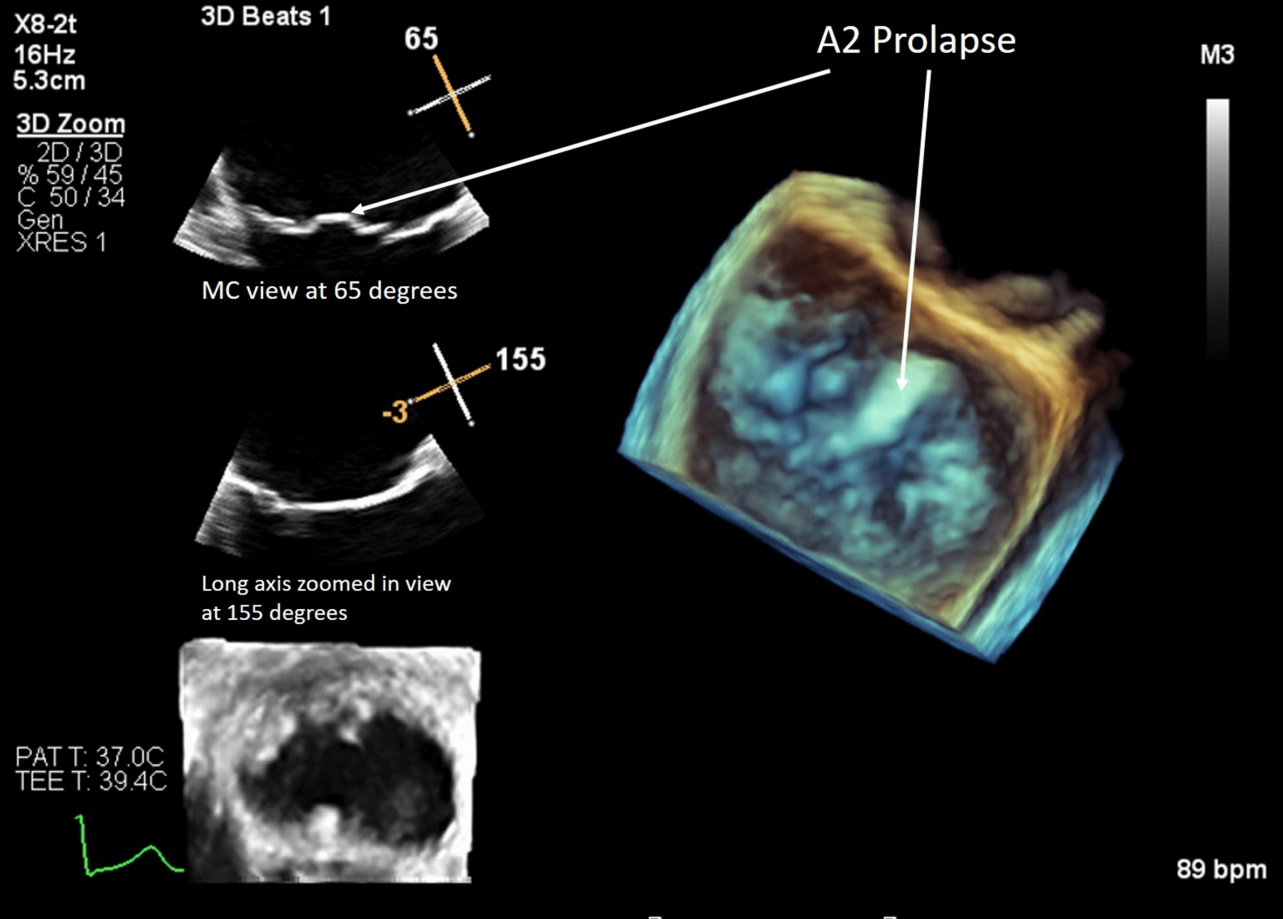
Three-dimensional TEE view of the mitral valve Three-dimensional TEE showing the mitral valve from the surgeon's view. The A2 scallop of the mitral valve is prolapsed as indicated by the white arrow. The angles of the TEE are indicated in the figure. TEE, transesophageal echocardiogram.
